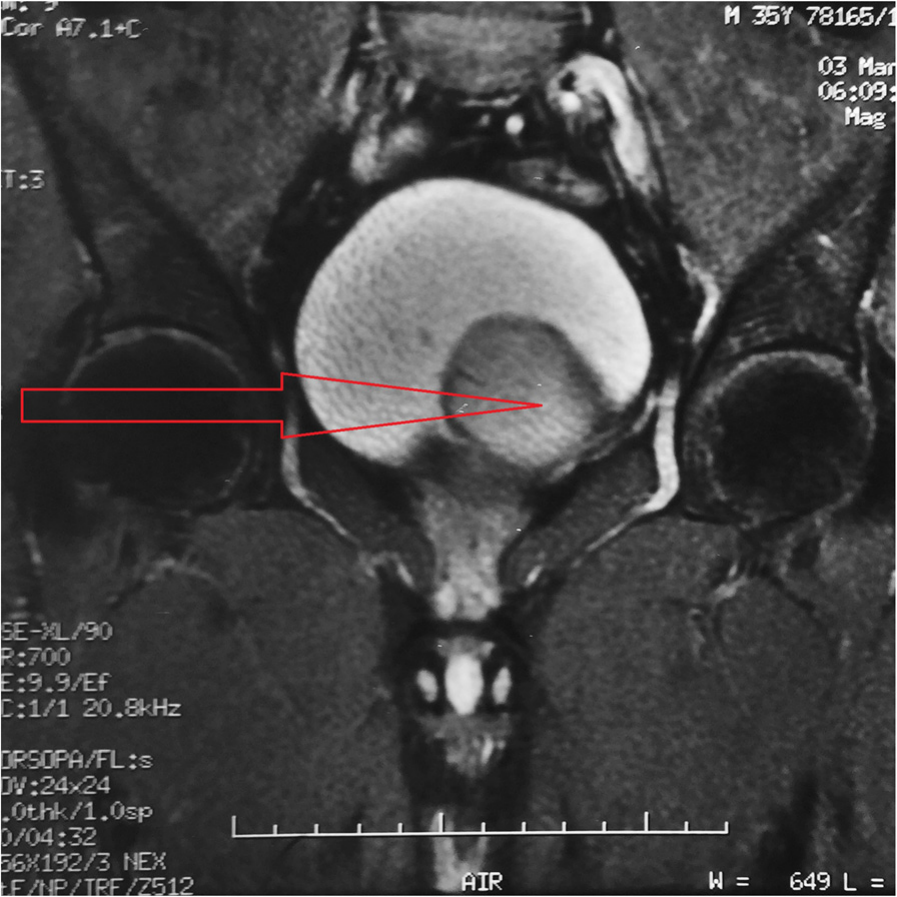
Pelvic MRI showing retrovesical cyst, limited training well lateralized left coarsely rounded (56 × 54 × 44) with thin wall
